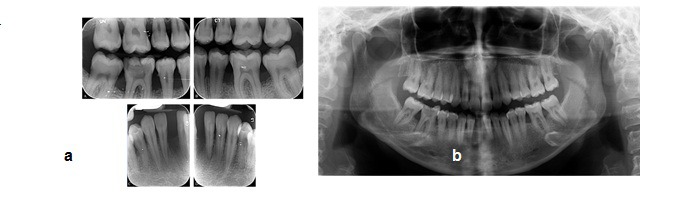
a Preoperative Panoramic radiograph  b Preoperative intraoral radiographs
